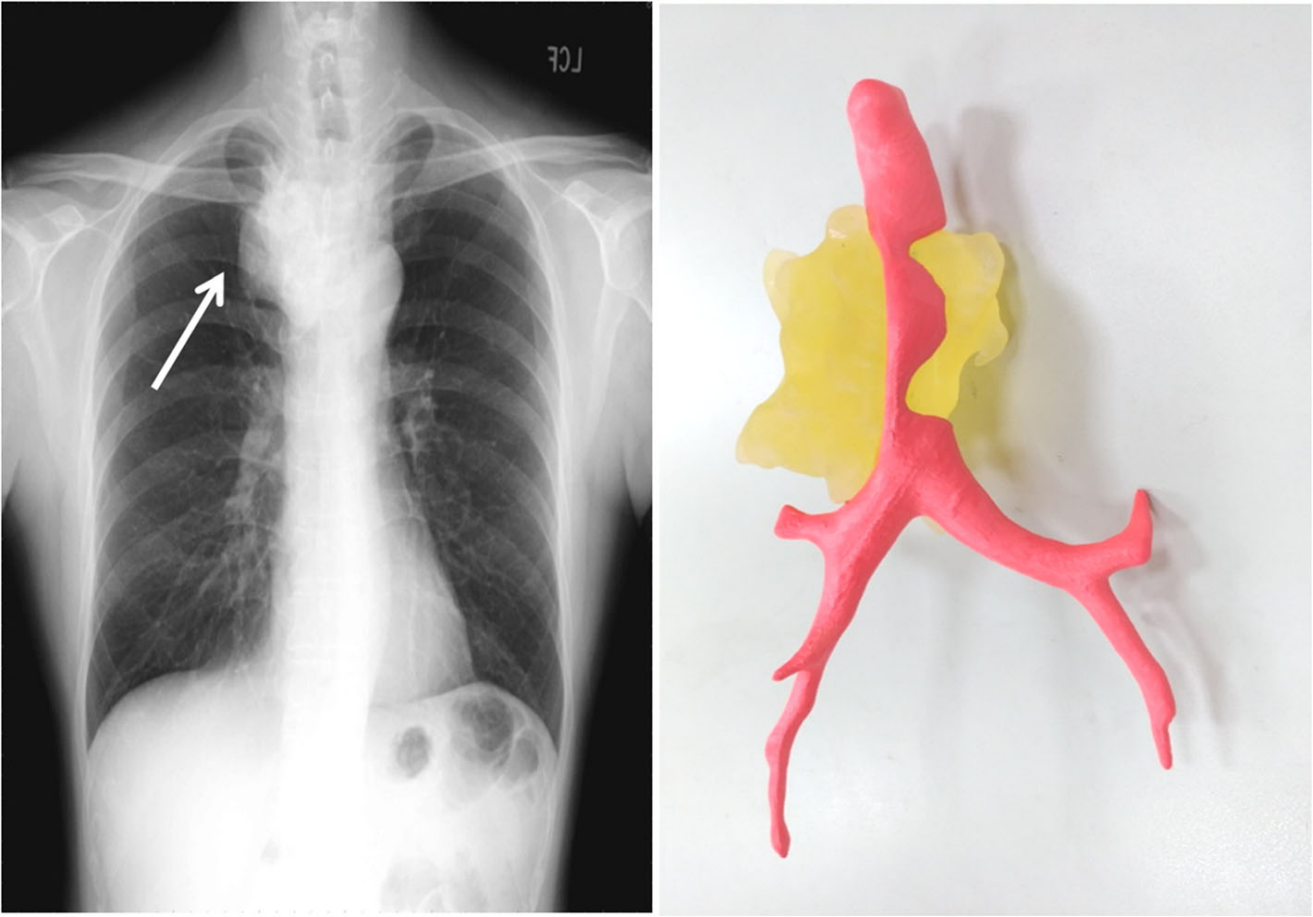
Chest x-ray and the 3-D model of the trachea and chondrosarcoma before surgery. *White arrow* marks the chondrosarcoma
